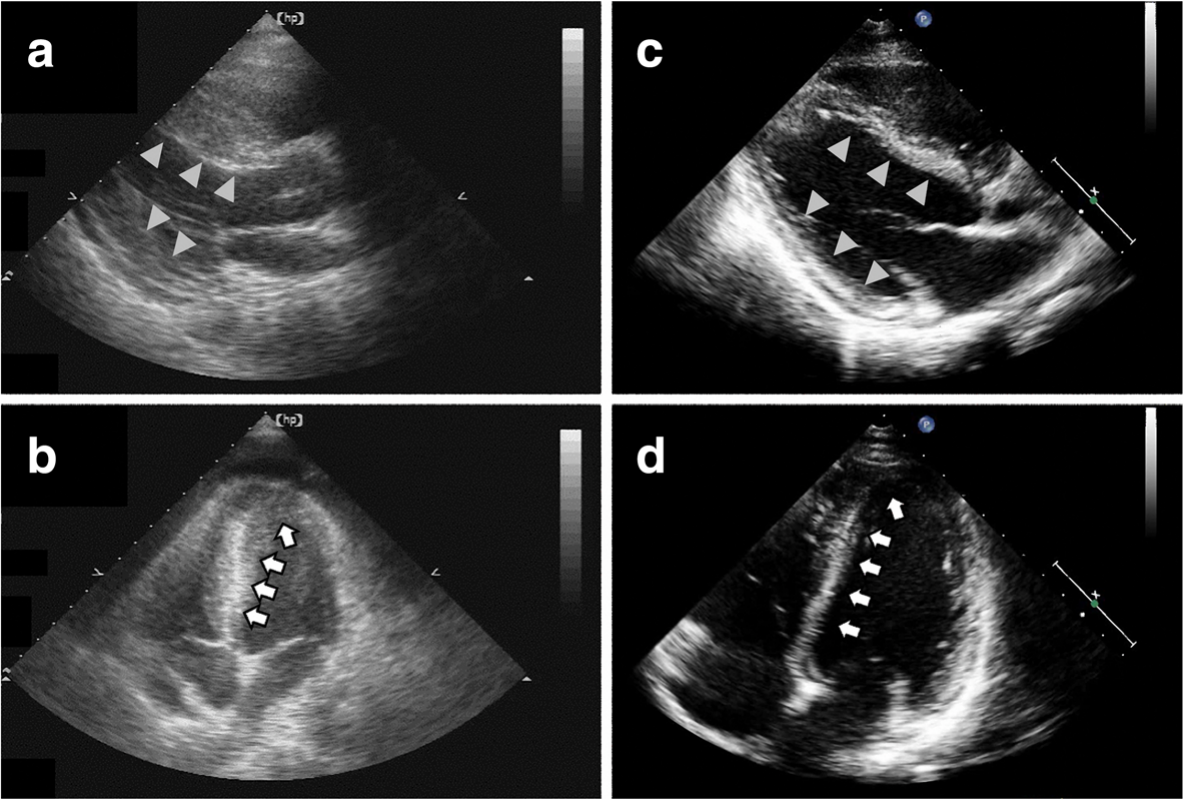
Transthoracic echocardiography showed dramatic improvement of left ventricle wall thickness and systolic motion after prednisolone treatment (10 mg) for 3 days. a Parasternal long axis view before treatment showed increased wall thickness of the septum and posterior wall (*arrowheads*). b Apical four chamber view before treatment showed increased wall thickness of the inferior septum, anterior lateral wall and apex (*arrows*). c Parasternal long axis view after treatment showed resolution of thickened wall of the septum and posterior wall (*arrowheads*). d Apical four chamber view after treatment showed resolution of the thickened inferior septum, anterior lateral wall and apex (*arrows*)
